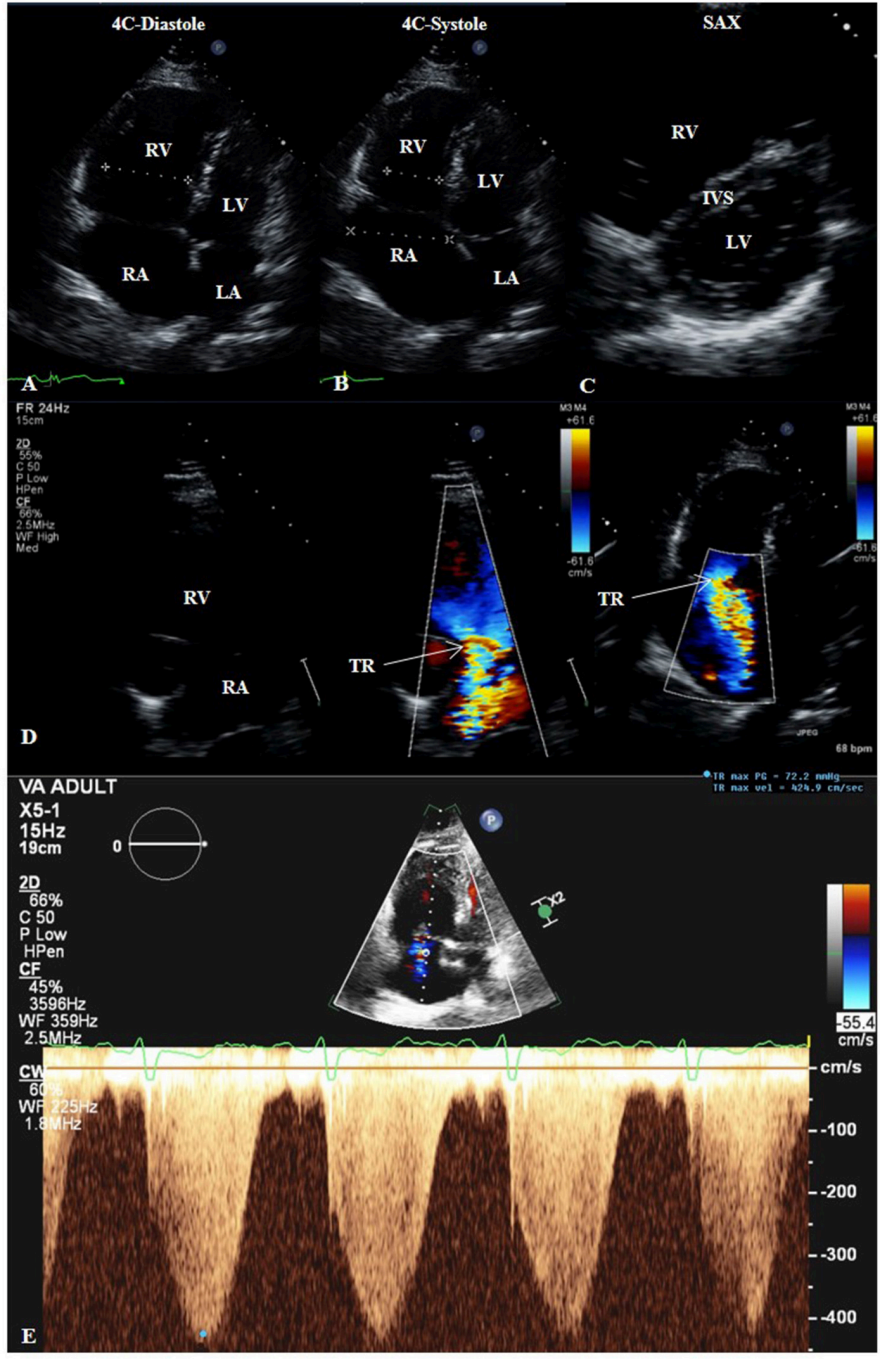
Transthoracic echocardiogram (TTE) of a patient with groups 2 and 3 pulmonary hypertension. 2D TTE in the apical four-chamber (4C) view in diastole (A) and systole (B) showing right heart dilatation. 2D TTE in the short-axis (SAX) view (C) showing flattening of the interventricular septum compatible with the right heart findings. (D) Color-compare TTE in the right ventricular inflow view and color Doppler TTE in the 4C view showing severe tricuspid regurgitation. (E) Continuous-wave Doppler TTE showing maximum trans-tricuspid valvular regurgitant jet velocity of 4.25 m/s, correlating to estimated pulmonary artery systolic pressure of 72 mmHg plus right atrial pressure. 4C, four-chamber; IVS, interventricular septum; LA, left atrium; LV, left ventricle; RA, right atrium; RV, right ventricle; SAX, short-axis; TR, tricuspid regurgitation.
